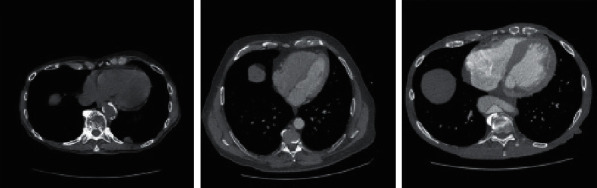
Some samples of RIDER Lung CT.
